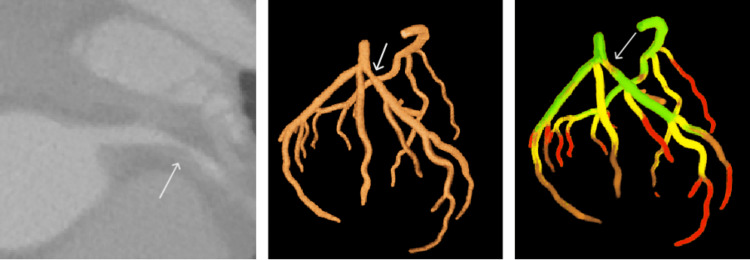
Left coronary artery with soft plaque in the ostium and the proximal segment of the LAD. (left) CT slice indicating a soft plaque (white arrow); (middle) 3D reconstruction of the coronary segment, emphasizing the plaque’s location (white arrow). (right) Clustering analysis, where the lower attenuation values of the plaque appear in the orange cluster (white arrow), indicating narrower, pathologic zones. LAD, left anterior descending artery
